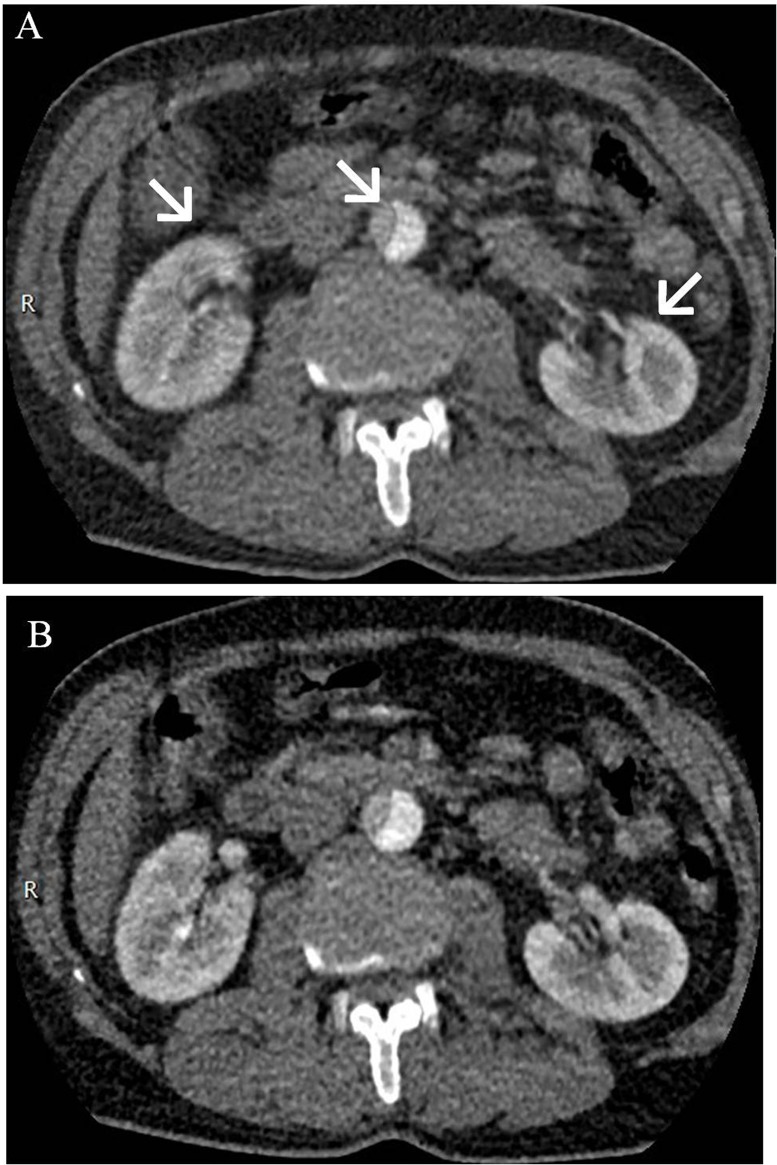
Transverse contrast-enhanced CT scan showed the effect of motion correction. (A) Motion artifacts were seen as blurring of the kidney and aorta contours (white arrowheads). (B) Most motion artifacts were not seen after correction.
